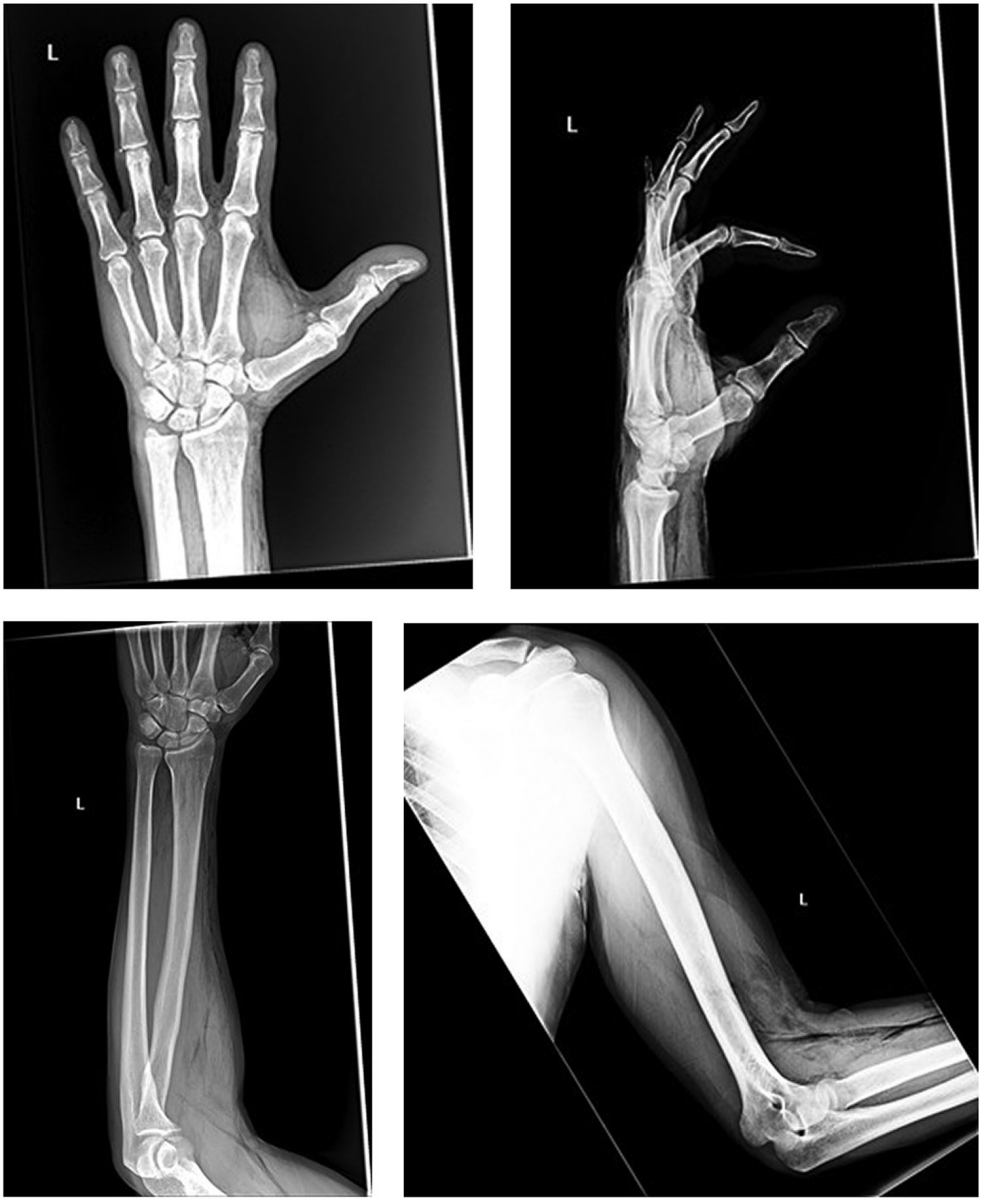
X-rays of left hand, wrist, forearm and humerus showing extensive subcutaneous emphysema throughout the hand, wrist, forearm, and arm.
